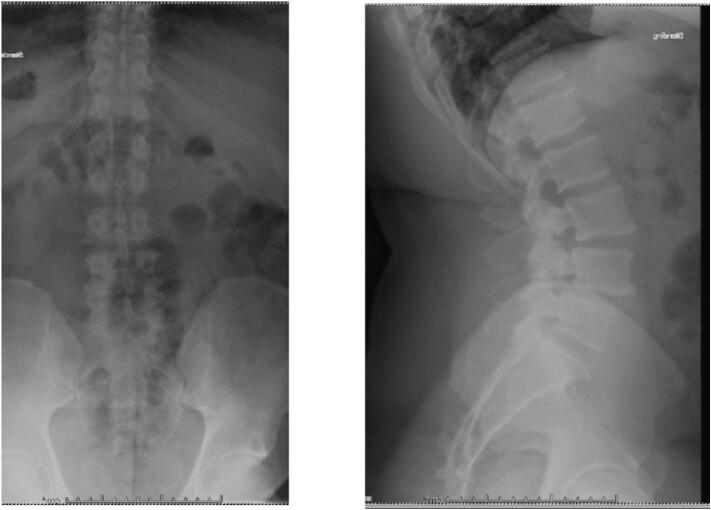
4/10/19 Preoperative Anterior/Posterior and Lateral Radiographs demonstrating L3–4, L4–5 isthmic spondylolisthesis.
